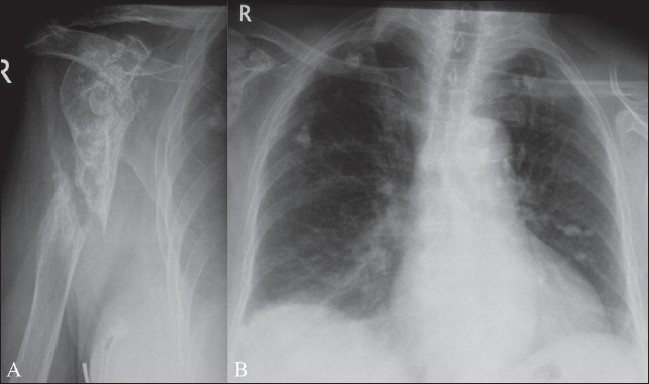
Pseudarthrosis of the humerus, as a complication of chronic tuberculous osteomyelitis at the site of a fracture. Plain radiograph of the right humerus (A) shows irregular sclerosis of the proximal humeral diaphysis and pseudarthrosis of the diaphysis. Note also partial destruction of the humeral head due to sequelae of associated tuberculous arthritis of the shoulder. Plain radiograph (B) of the chest reveals bilateral calcified granulomas within the lungs
